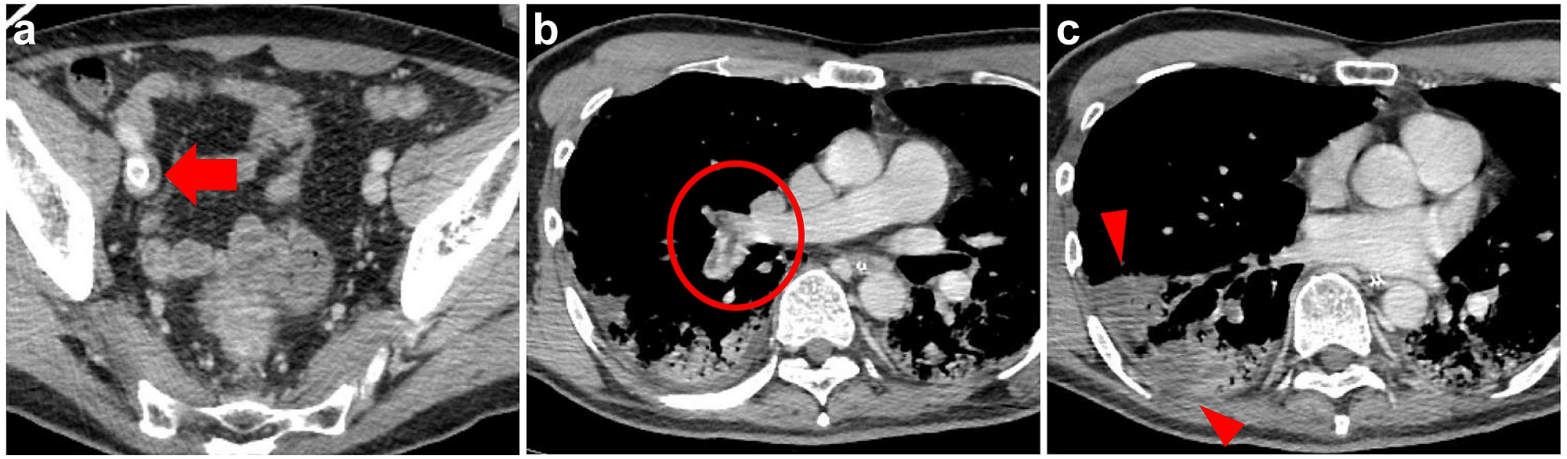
Chest-pelvis contrast-enhanced computed tomography images (a, b) Deep vein thrombosis of the right iliac vein around the drainage cannula for extracorporeal membrane oxygenation (arrow) and pulmonary embolism of the right main pulmonary artery (circle). (c) Pulmonary infarction, most likely secondary to a pulmonary embolism owing to the presence of poor contrast over a wedge along the embolized pulmonary artery region (arrowhead).
